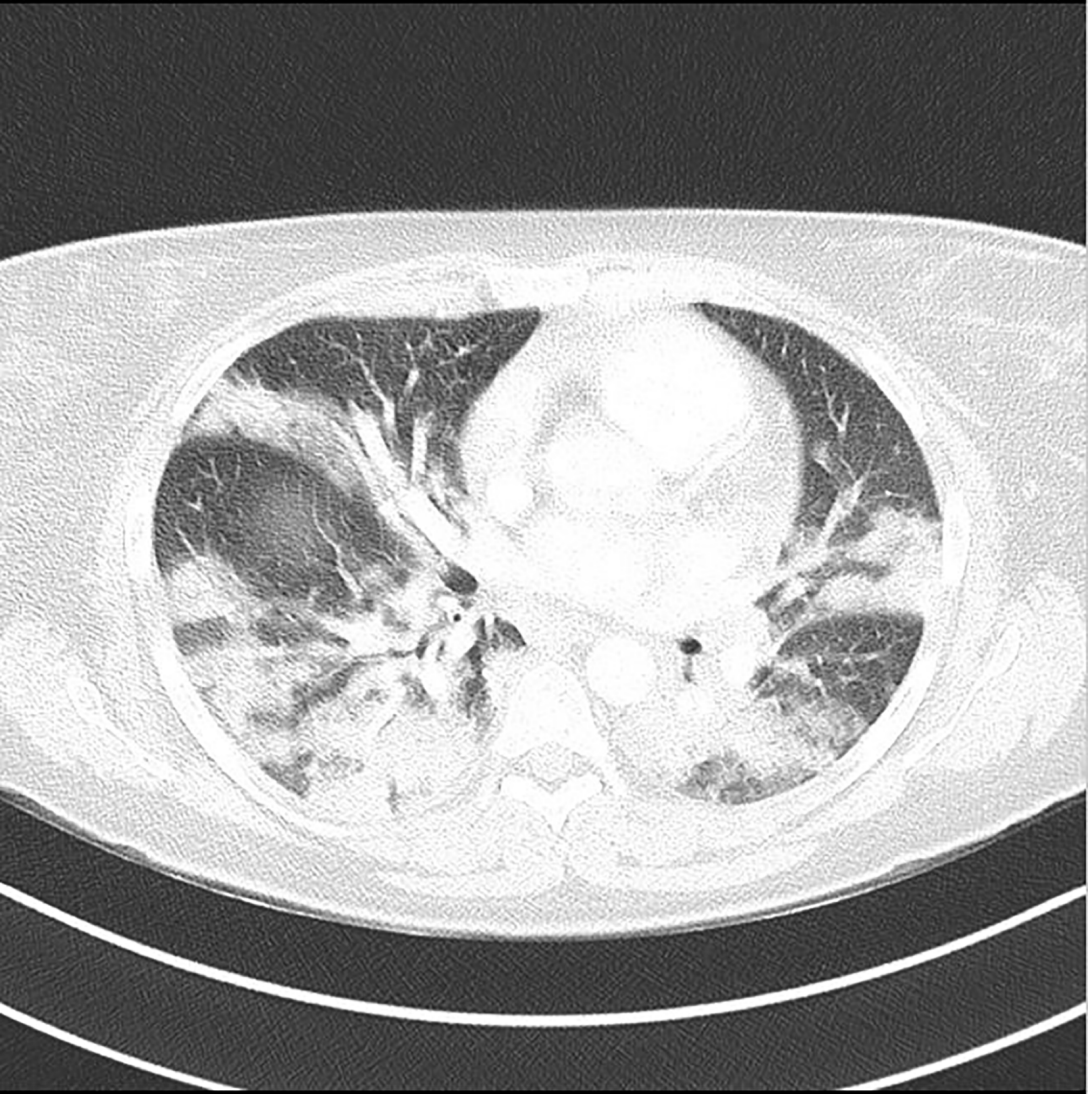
Chest CT showing signs of acute pulmonary embolism, along with extensive ground-glass opacifications involving both the lung parenchyma.
